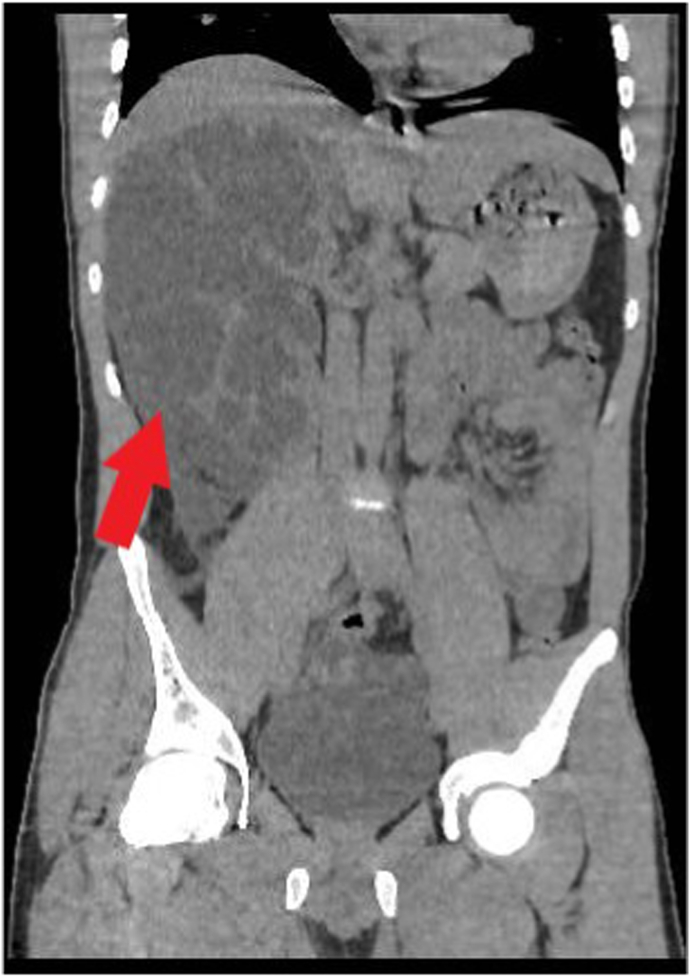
Coronal section of CT abdomen showing perinephric collection (red arrow). (For interpretation of the references to colour in this figure legend, the reader is referred to the Web version of this article.)
